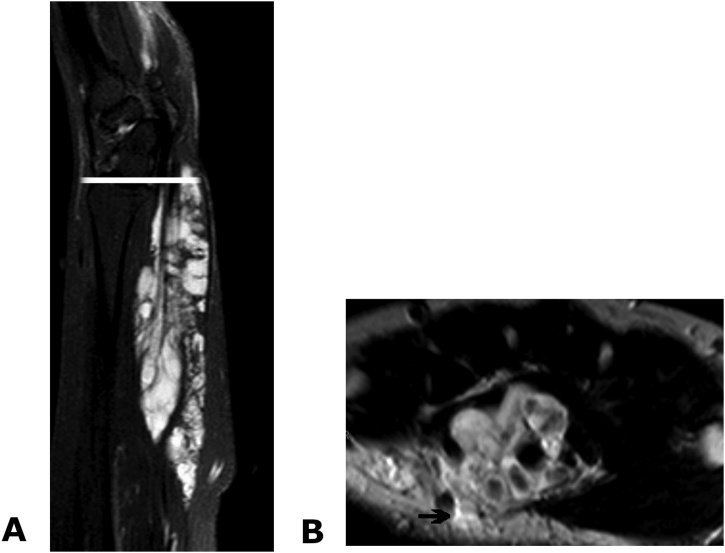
MRI showing expansion of the venous malformation in the carpal tunnel, resulting in compression of the median nerve (arrow). Left, sagittal view; right, axial view at the level of the bar in the left image.
